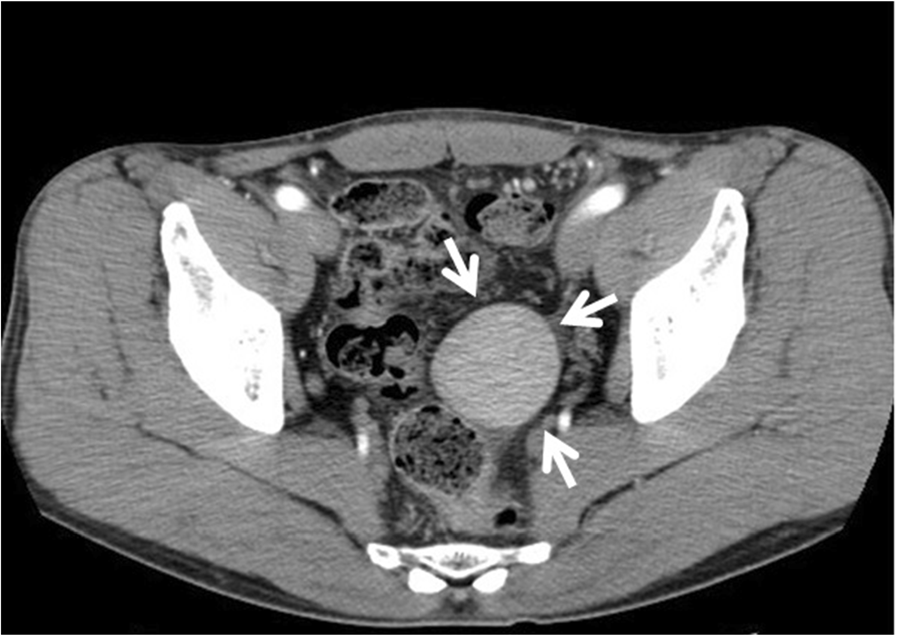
The pelvic mass on dynamic computed tomography. A 40-mm solid pelvic mass was observed anterior to the rectum and was enhanced homogeneously (*arrow*)
